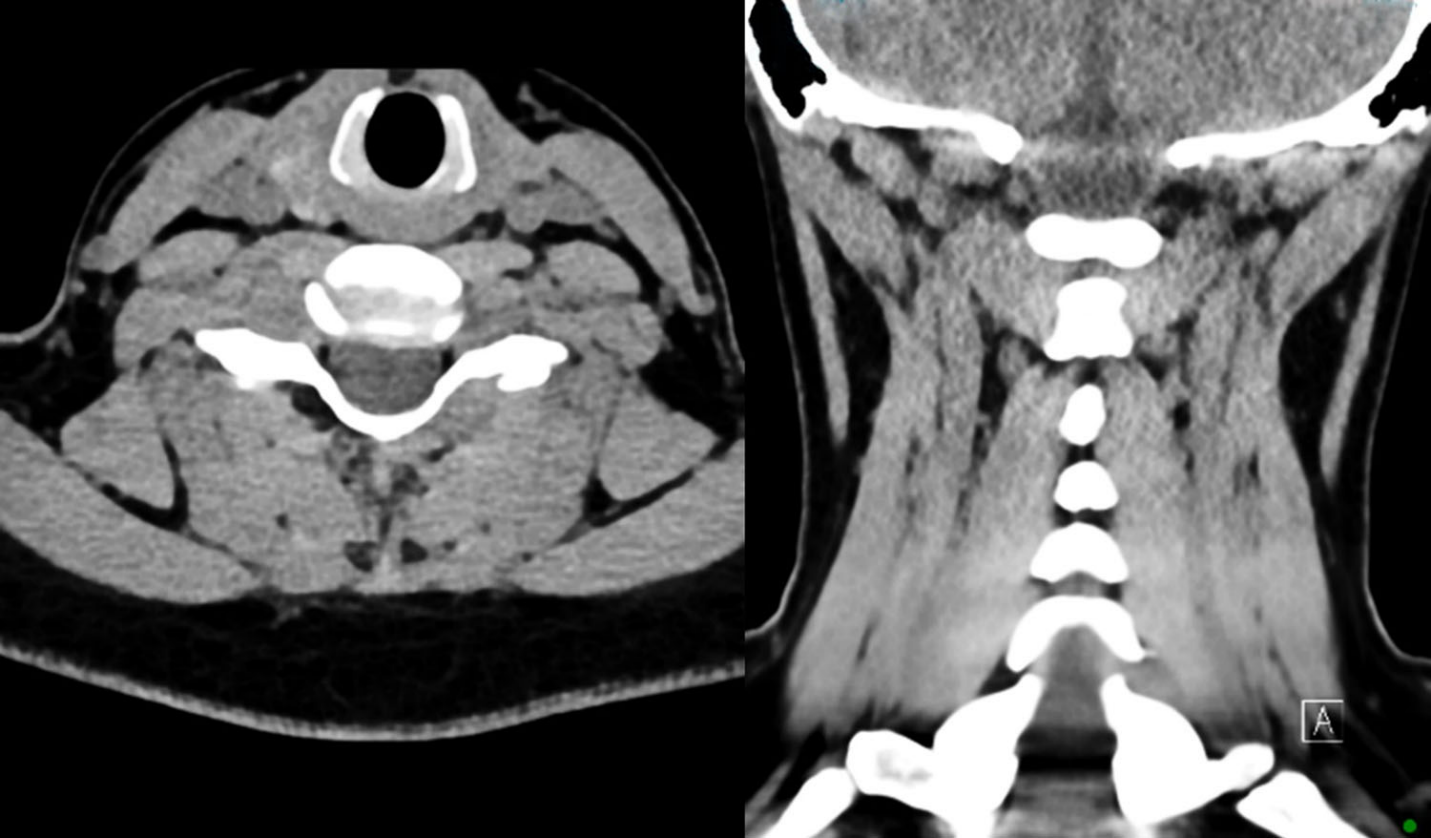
Case 2 CT scan. There was a soft tissue density focus at the left C7 lamina that extended to the medial aspect of the left C6/7 facet joint and measured 2 × 0.5 × 1.6 cm. There was bony remodeling of the left C6/7 articular column without overtly aggressive features.
